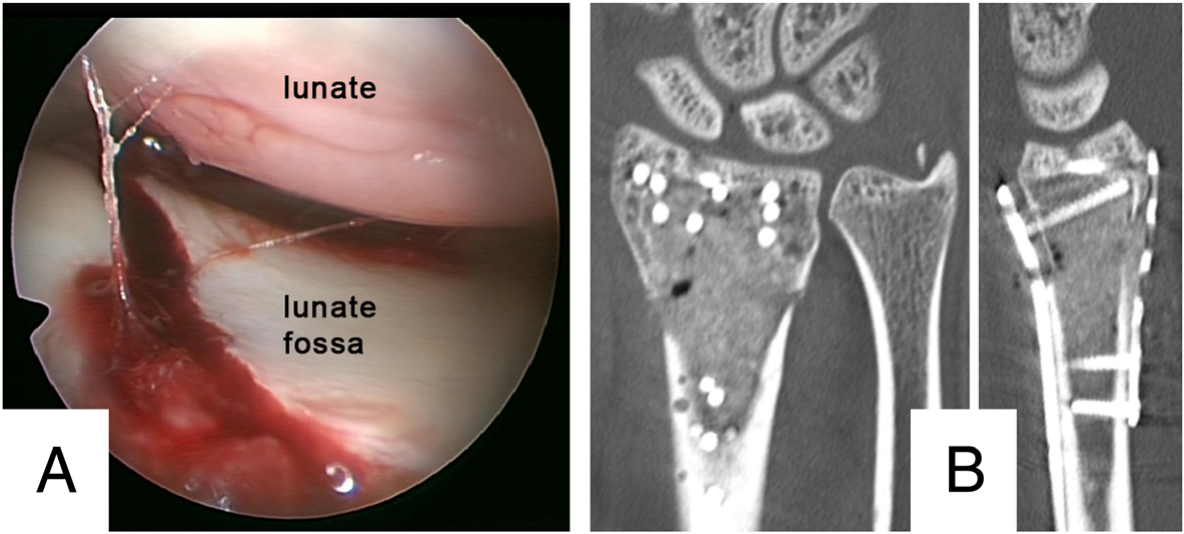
Perioperative arthroscopic images and postoperative CT-scan. A) Perioperative image of the arthroscopy showing the articular surface after mobilization and repositioning of the ulnar and radial fragment; B) postoperative CT scan with good joint alignment.
